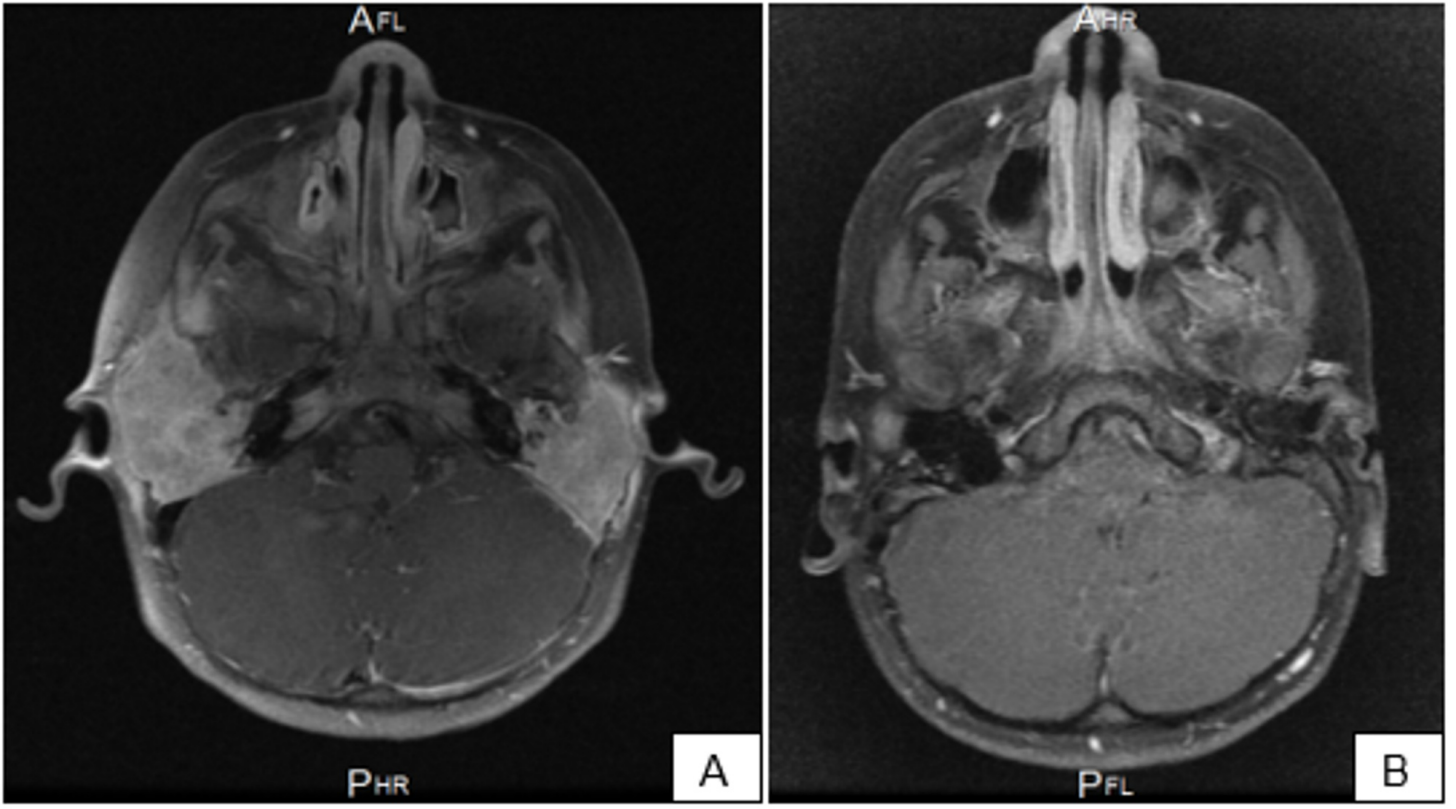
Axial view of post-contrasted T1 MRI images A: the image shows the heterogeneous enhancing lesion in the temporal bone area bilaterally; B: the image shows the decreased enhancement of the temporal bone lesions post-salvage protocol MRI: magnetic resonance imaging
